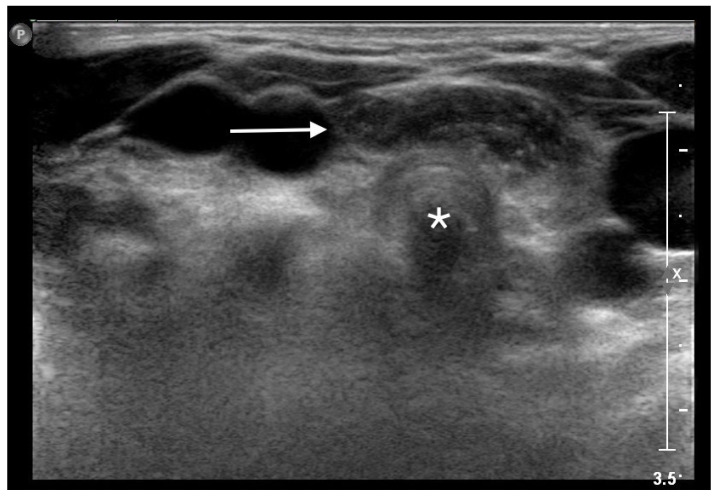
Superior herniation of the normal mediastinal thymus in a 5-year-old girl. Transverse sonogram obtained during the Valsalva maneuver showing a roughly oval structure (*white arrow*) with the typical “starry sky” appearance of thymus, in front of the cervical trachea (*white star*).
